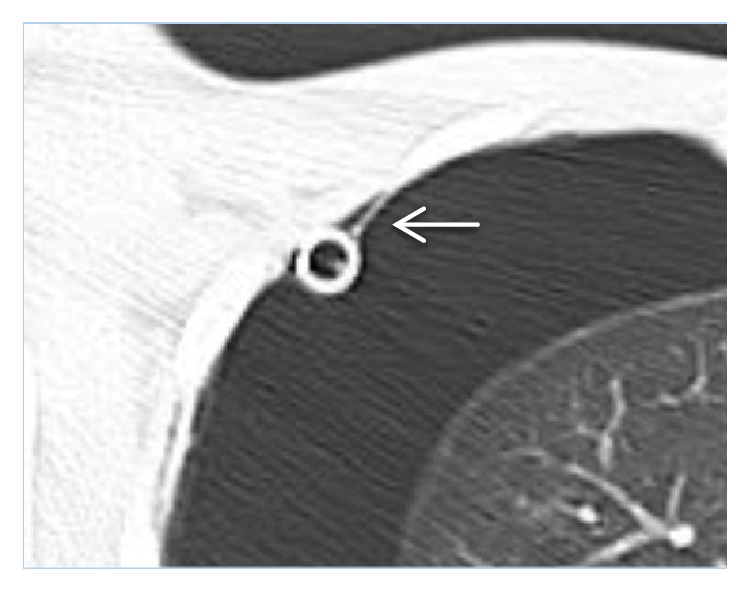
Chest CT axial cut magnified showing the location of the chest tube in relation to the chest wall and parietal pleura. White arrow: parietal pleural.
